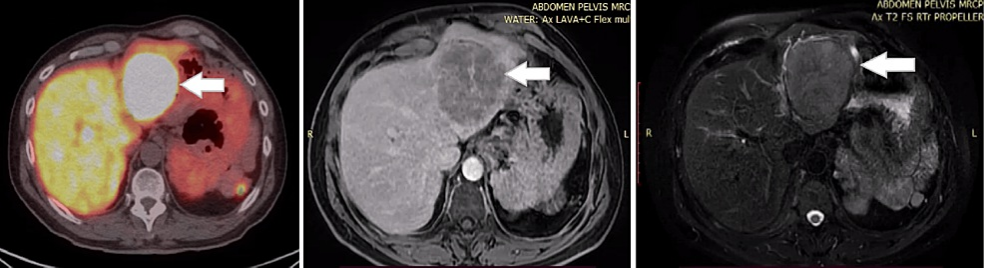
Axial PET-CT and MRI images of the abdomen The white arrow demonstrates a left lobe liver lesion. PET-CT: positron emission tomography-computed tomography; MRI: magnetic resonance imaging
